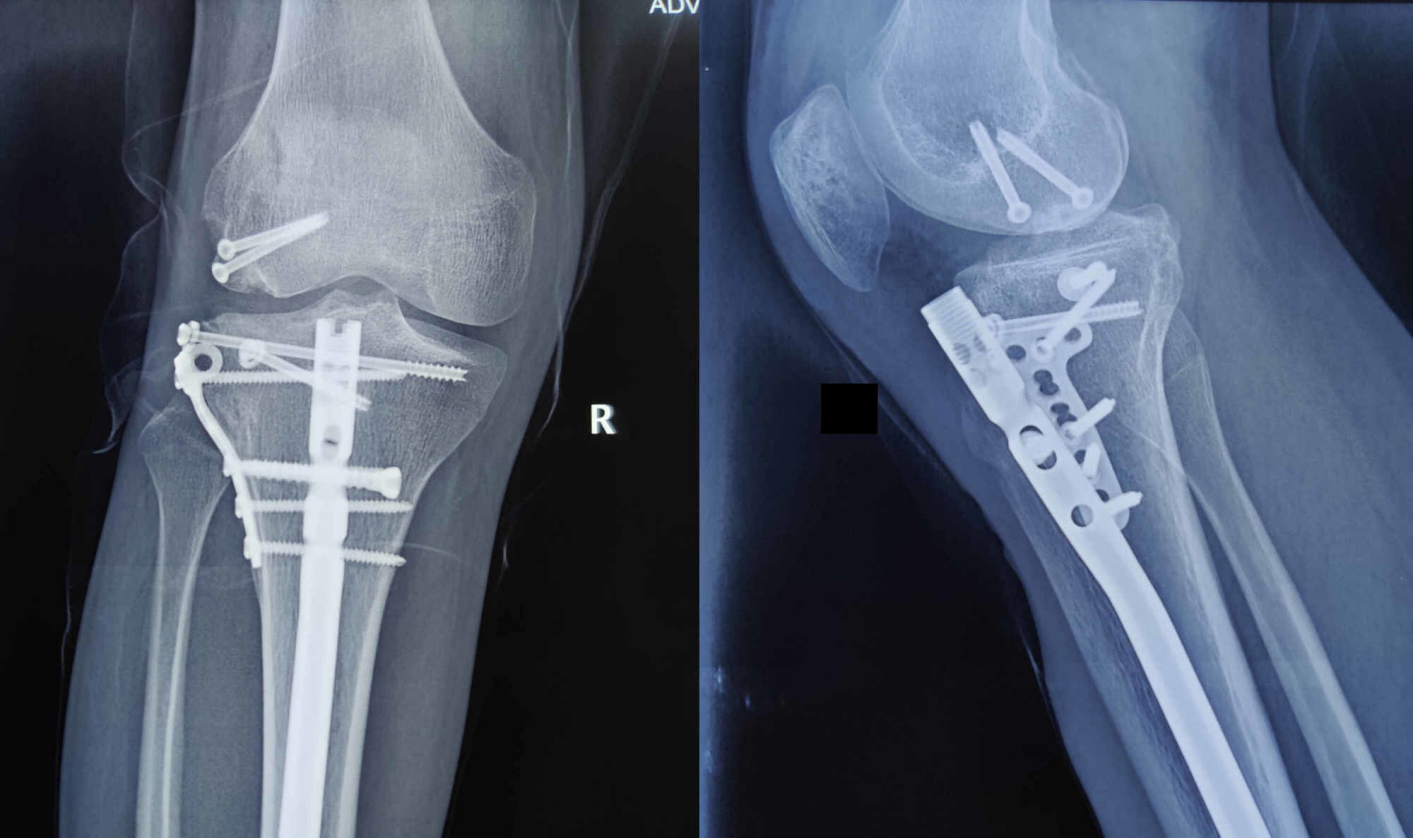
Anteroposterior and lateral radiographs of the knee at one-year follow-up
